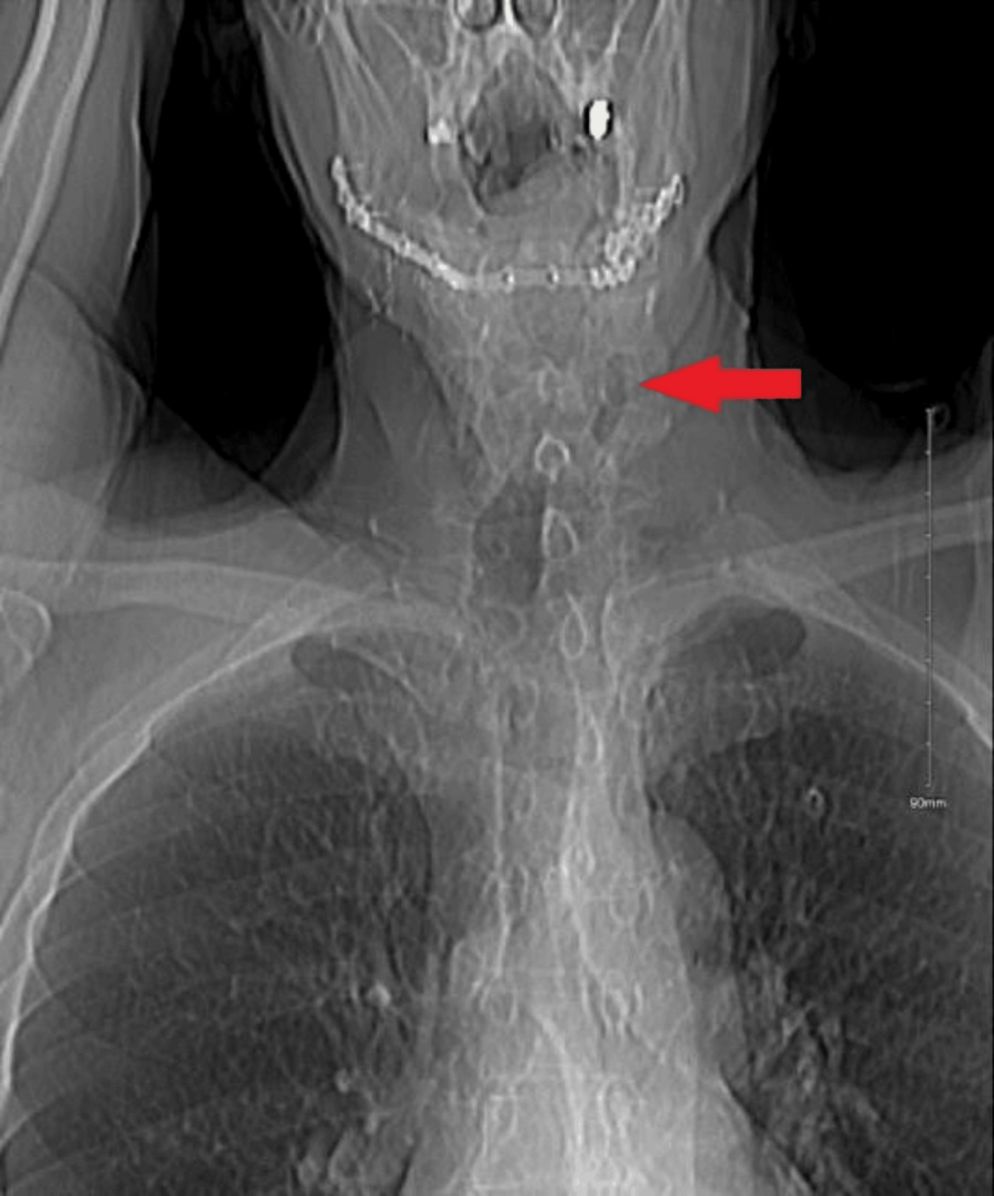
X-ray chest: red arrow pointing to left-sided deviation of the trachea.
